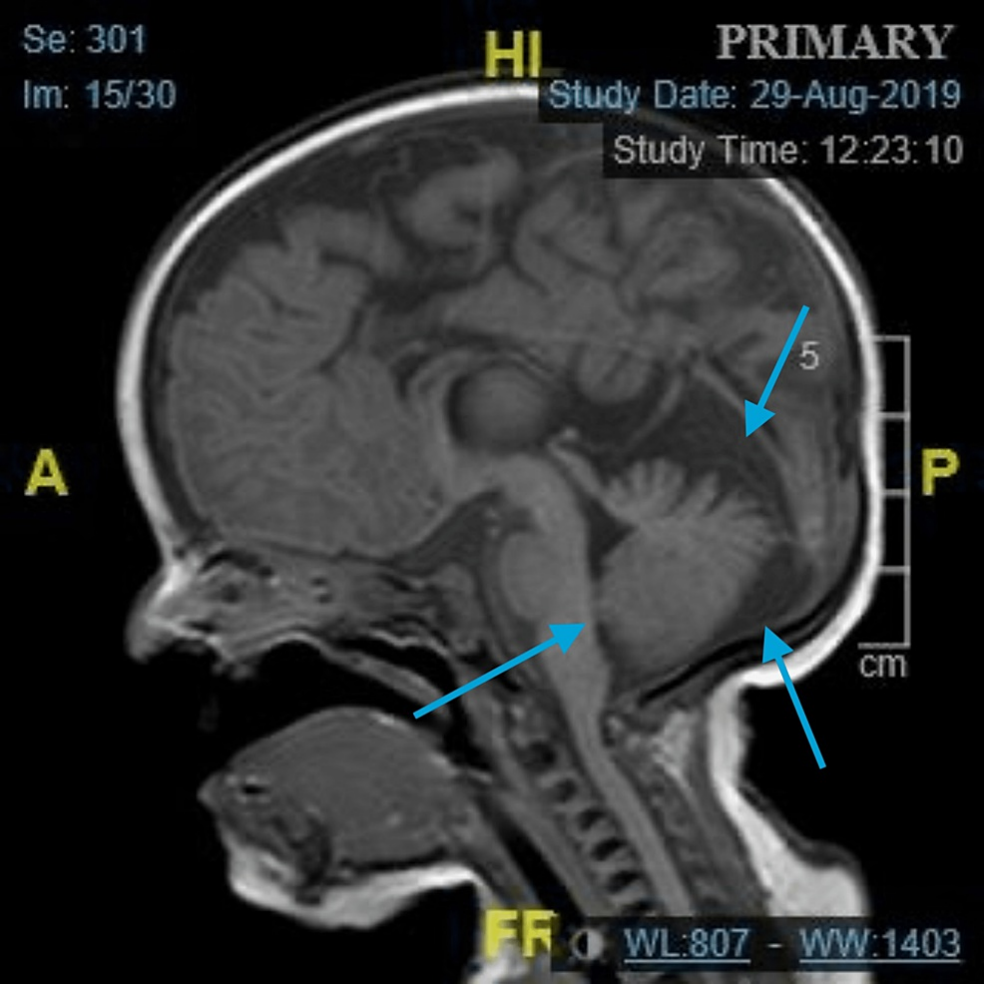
Sagittal section of T1-weighted MRI with vermian hypoplasia, shifting of the fastigium, and deep interpeduncular fossa (blue arrows). MRI: magnetic resonance imaging, A: anterior, P: posterior, Hl: head length, FR: frequency, CM: centimeter
